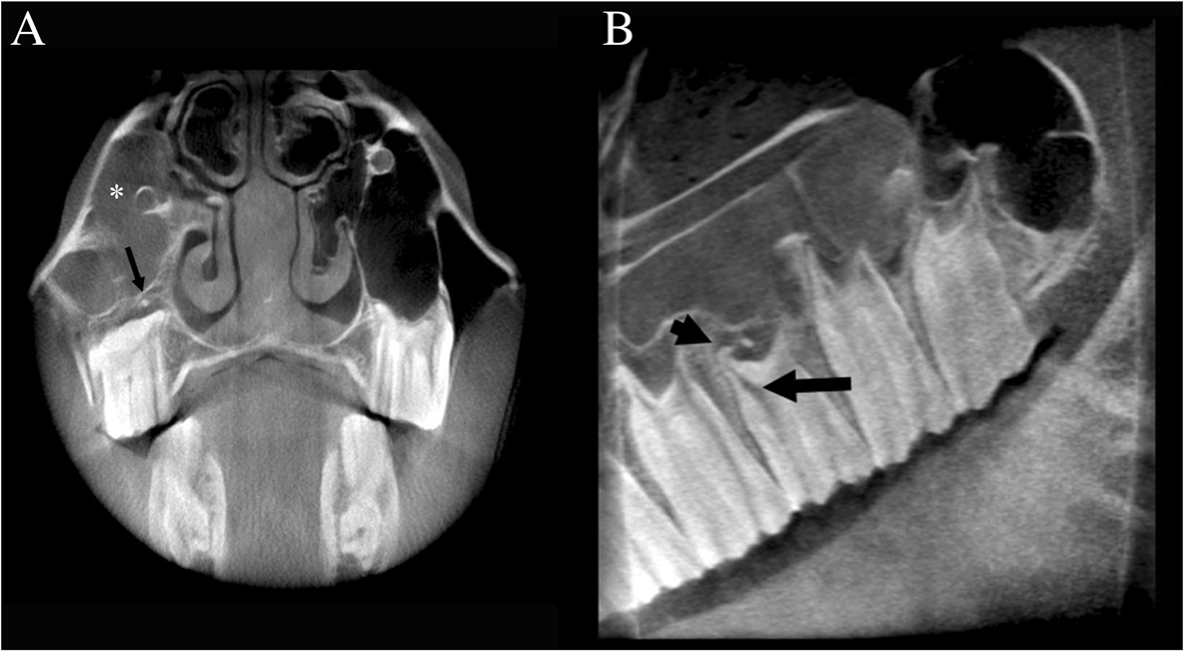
Thirteen-year-old Warmblood gelding with an apical infection of the tooth 109. a Transverse CBCT image at the level of 109; note the soft tissue attenuating material (asterisk) filling the rostral maxillary sinus and the presence of a small cementoma in the alveolar space at the level of the palatine tooth root (long black arrow). b Sagittal CBCT image through the right maxillary arcade: note the widening of the rostral pulp canal and the clubbing of the palatinal tooth root
